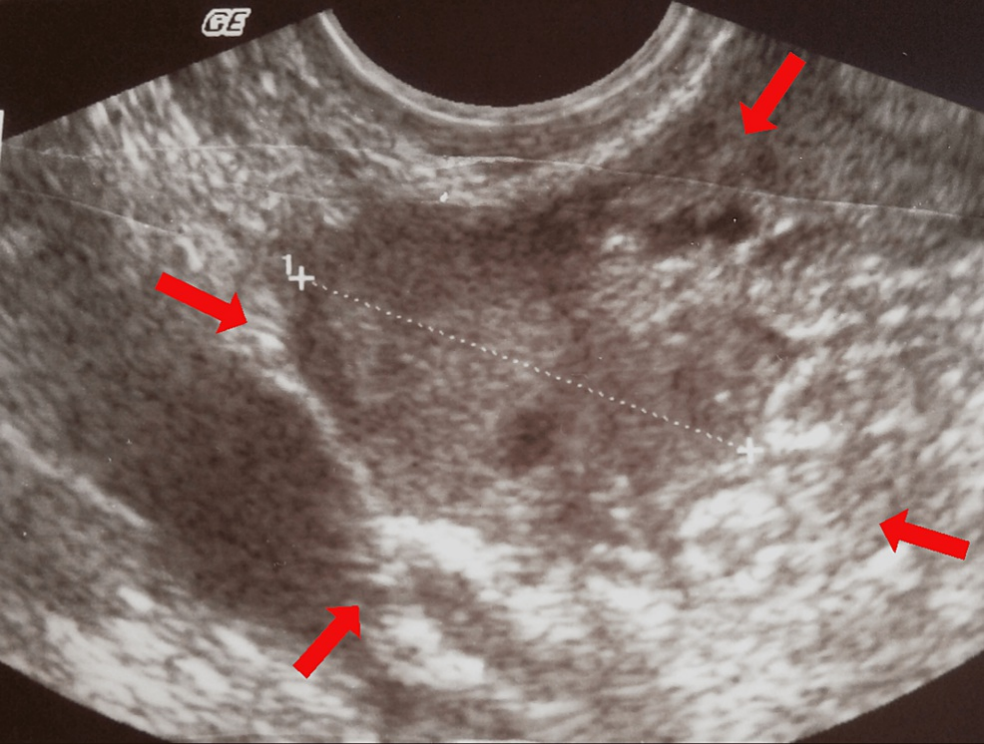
Transvaginal ultrasound imaging of an ectopic ovarian pregnancy (our case) The presence of heterogeneity in the anatomical area of the adnexa (red arrows), combined with the absence of an intrauterine gestational sac, supports the diagnosis of ruptured ectopic pregnancy.
